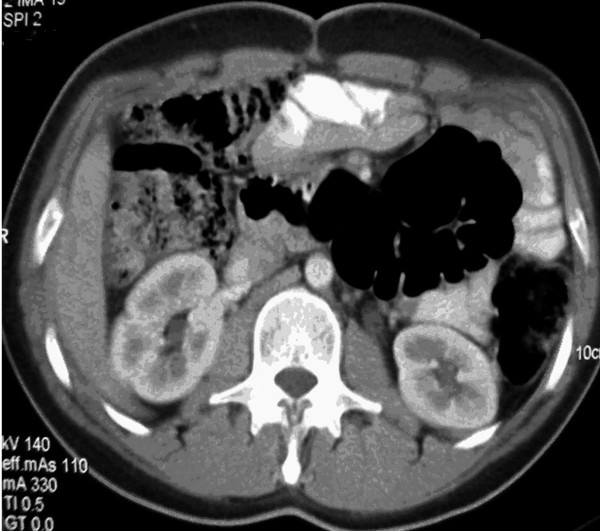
CT scan. No evidence of tumor activity is demonstrated. Localization of intraabdominal organs is adequate.
